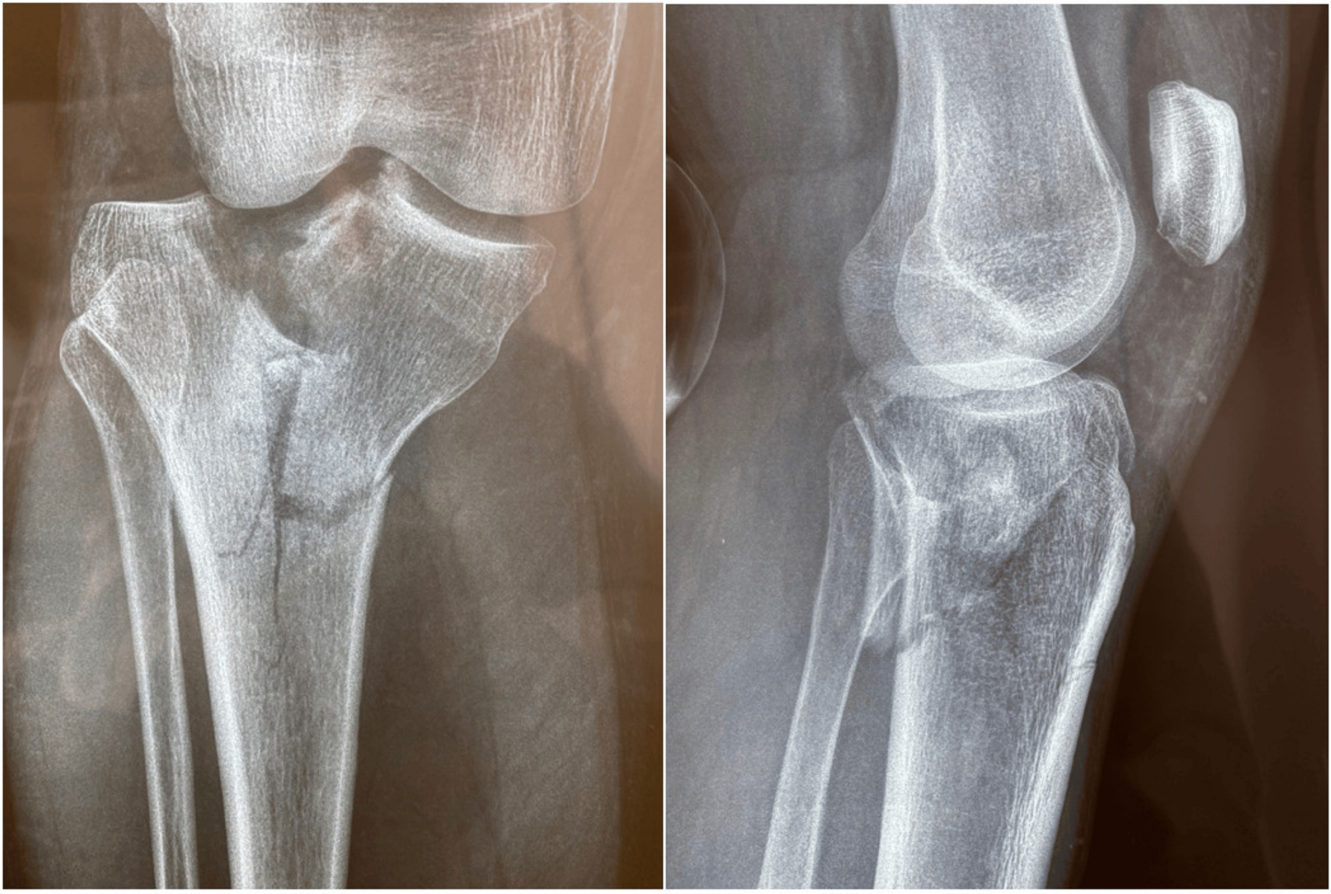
Preoperative X-rays of a patient with a Schatzker type VI tibial plateau fracture
